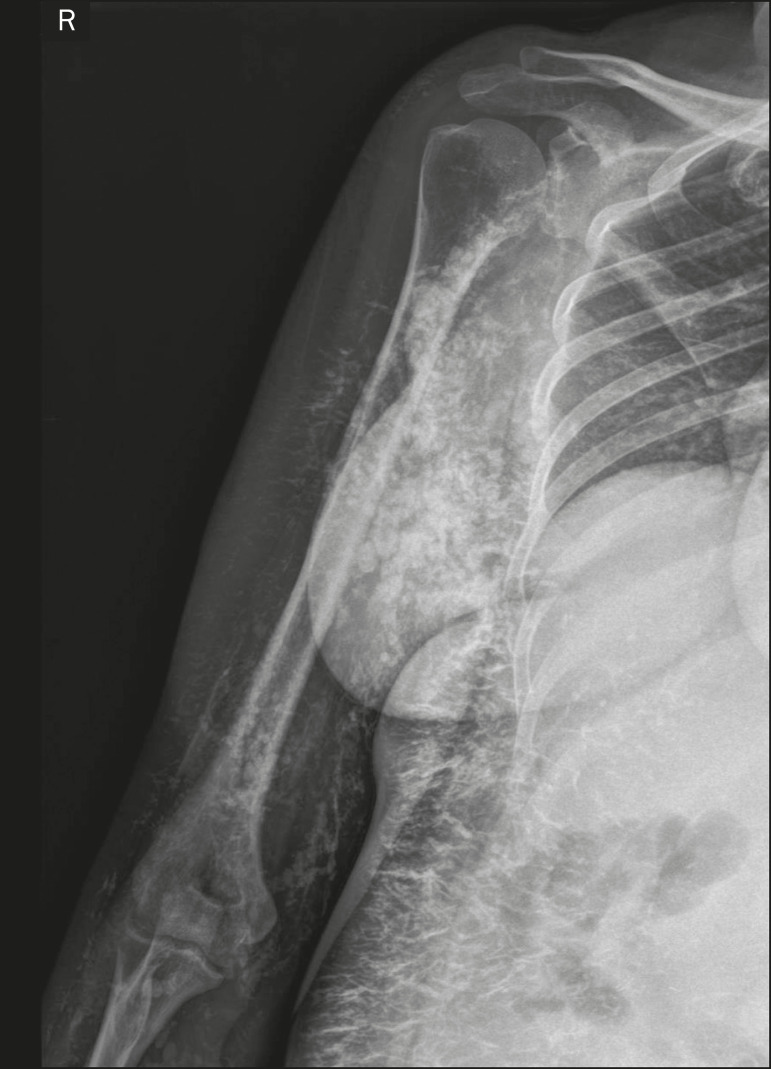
Calcinosis universalis in dermatopolymyositis. Frontal X-ray of the shoulder and arm showing diffuse calcifications in the skin, subcutaneous tissue, fasciae, and muscles. Note the leaf-like distribution of the calcifications.
